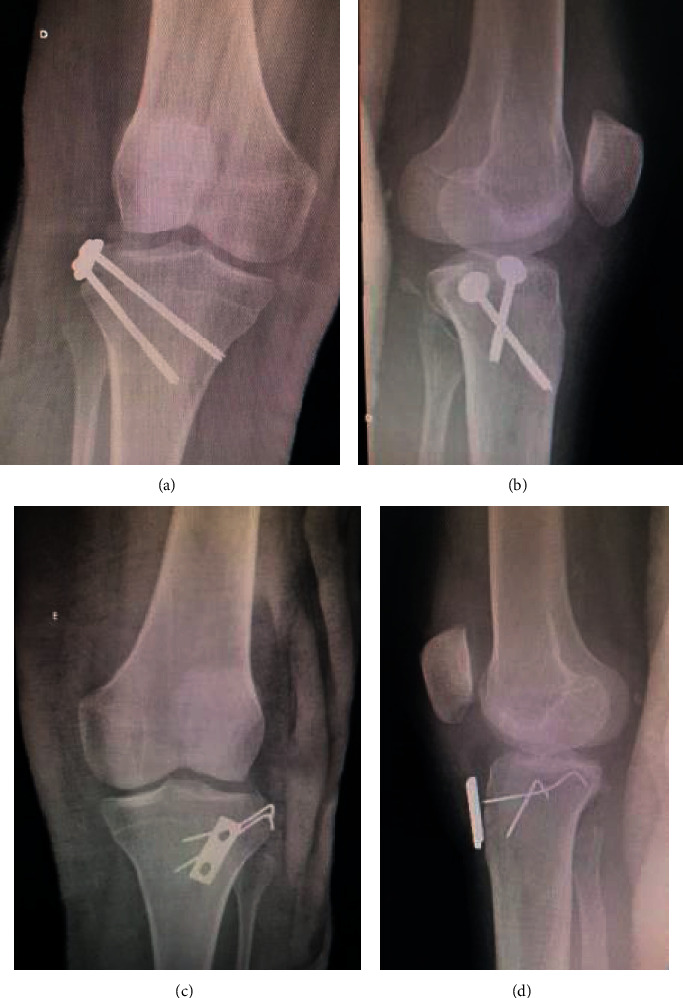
Anteroposterior radiographs pos operative and lateral view of the right knee (a, b) and left knee (c, d).
